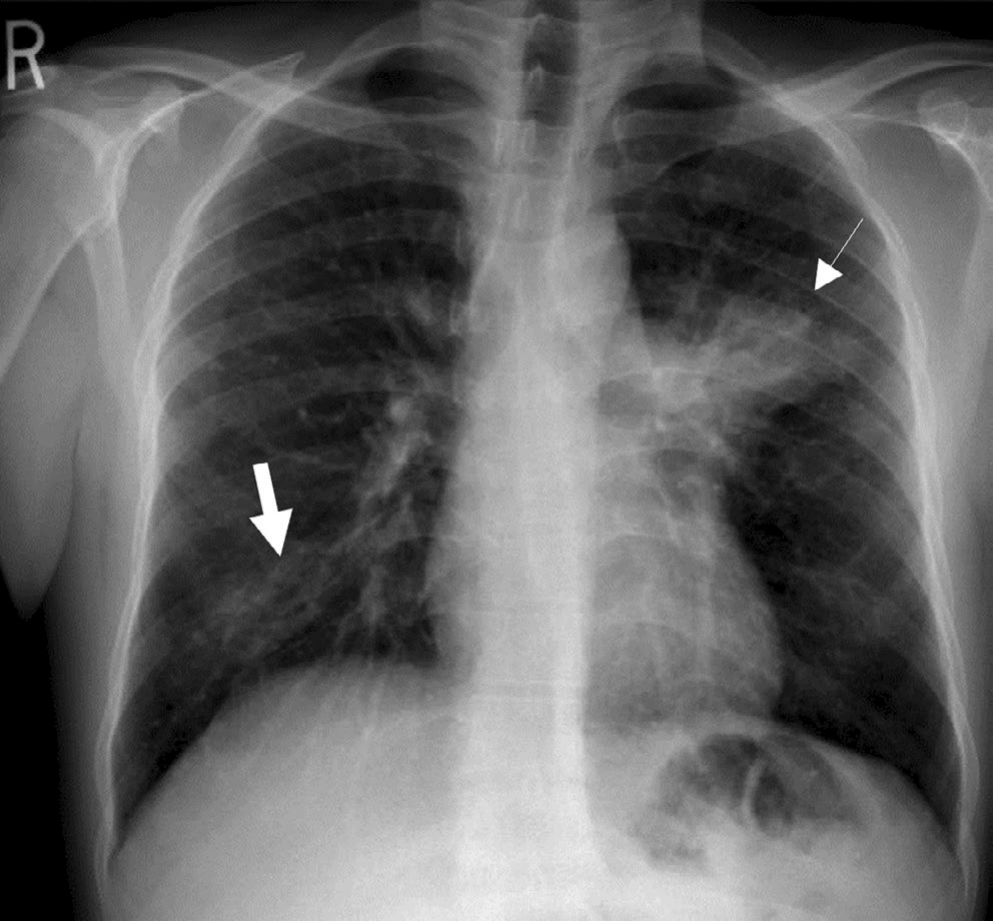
Postero‐anterior radiograph of the chest showing left peri‐hilar patchy consolidation with (thin white arrow) with areas of patchy consolidation at the right lower zone (thick white arrow).
